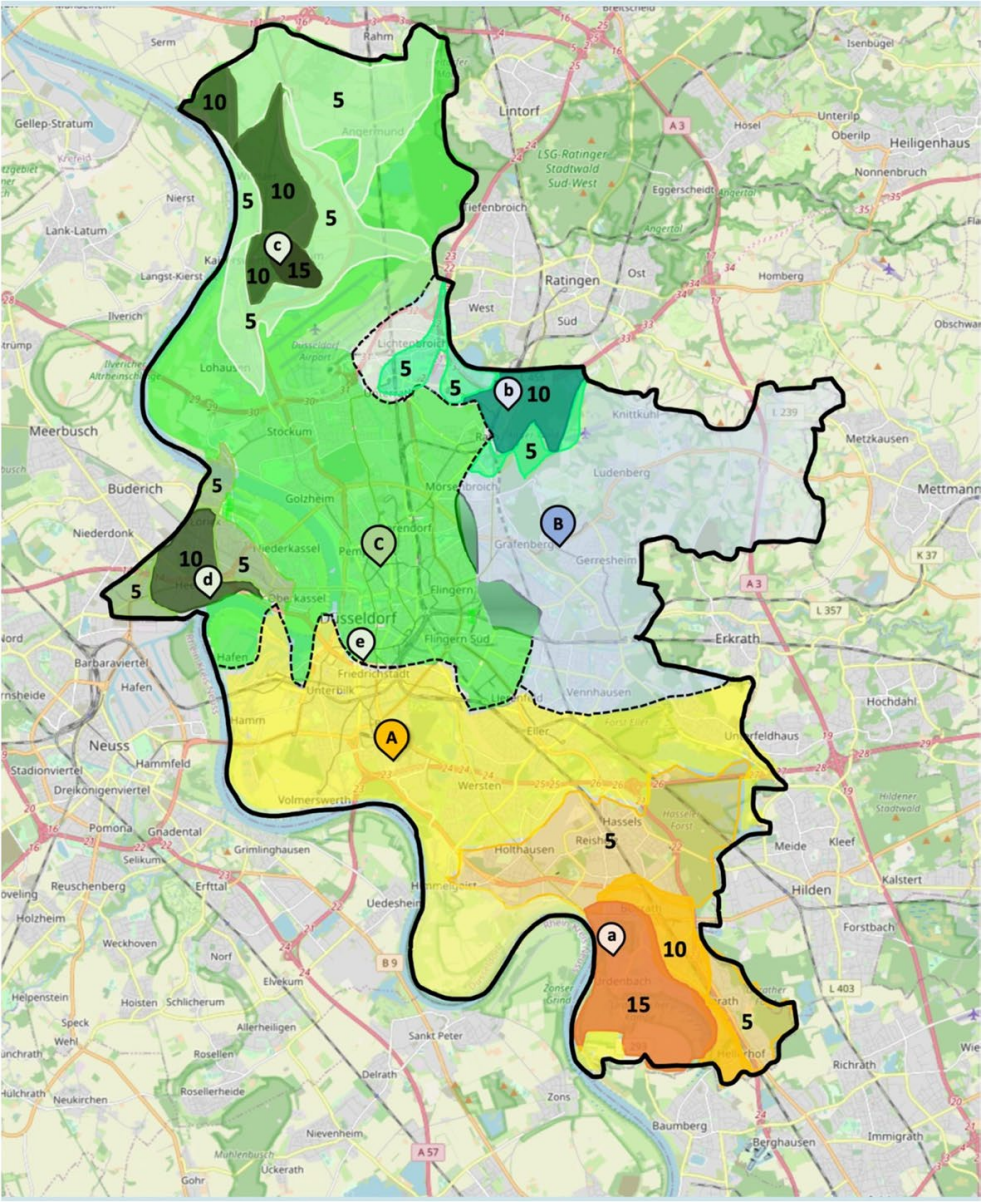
Isochrone maps for transport times for all stroke units. A comprehensive stroke center (yellow); B (blue), C (green) = primary stroke centers, a-d = general hospitals; numbers indicate the time benefit of transporting patients to the nearest CT (a-e) rather than the Stroke Unit in minutes
